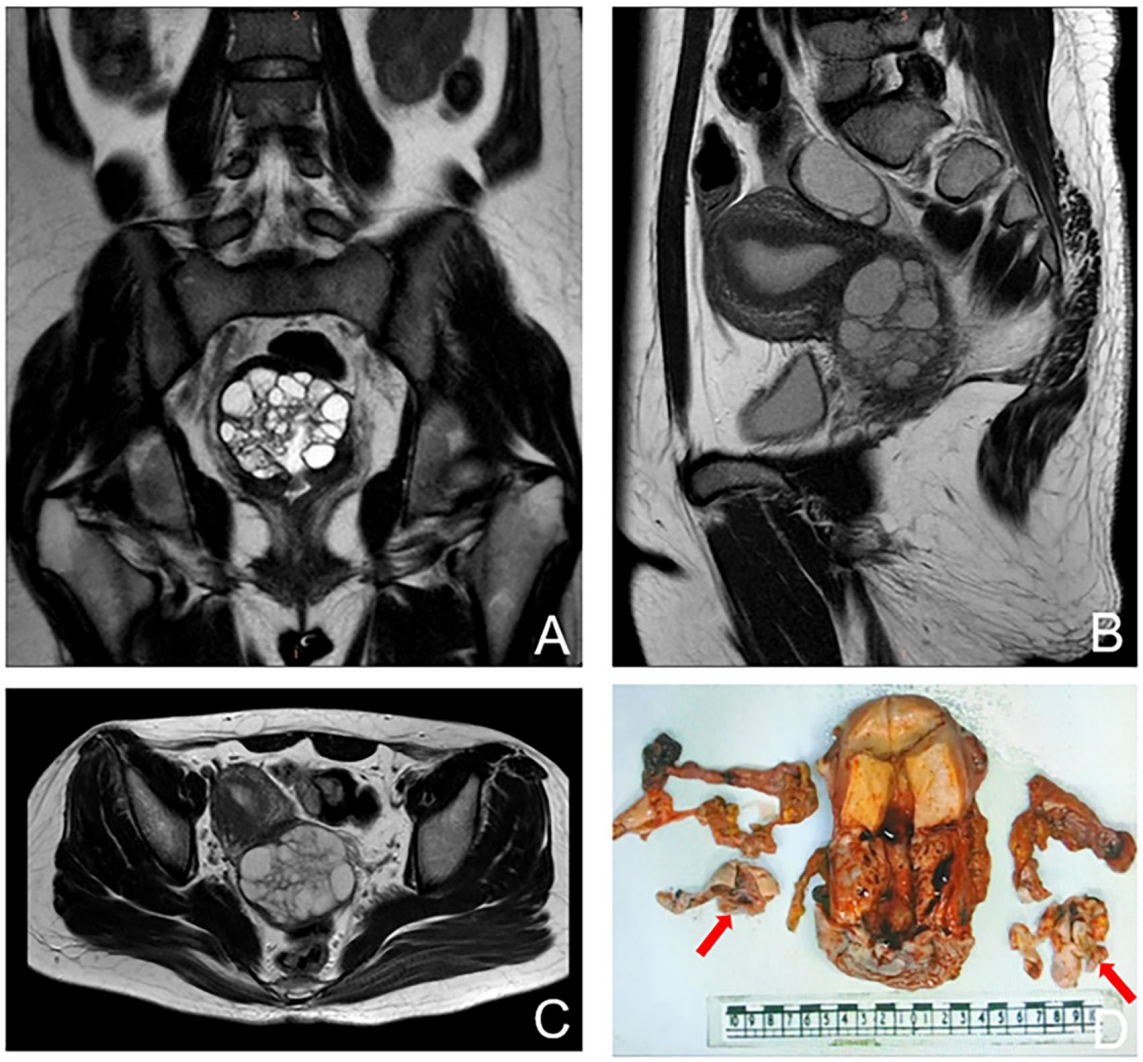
Magnetic resonance imaging (MRI) images showing an enlargement of the cervix with multiple cystic lesions. (A) coronal plane. (B) sagittal plane. (C) transverse plane. (D) Gross features of the uterus showing bulging cervix, fallopian, and ovary. The arrows show bilateral ovaries.
